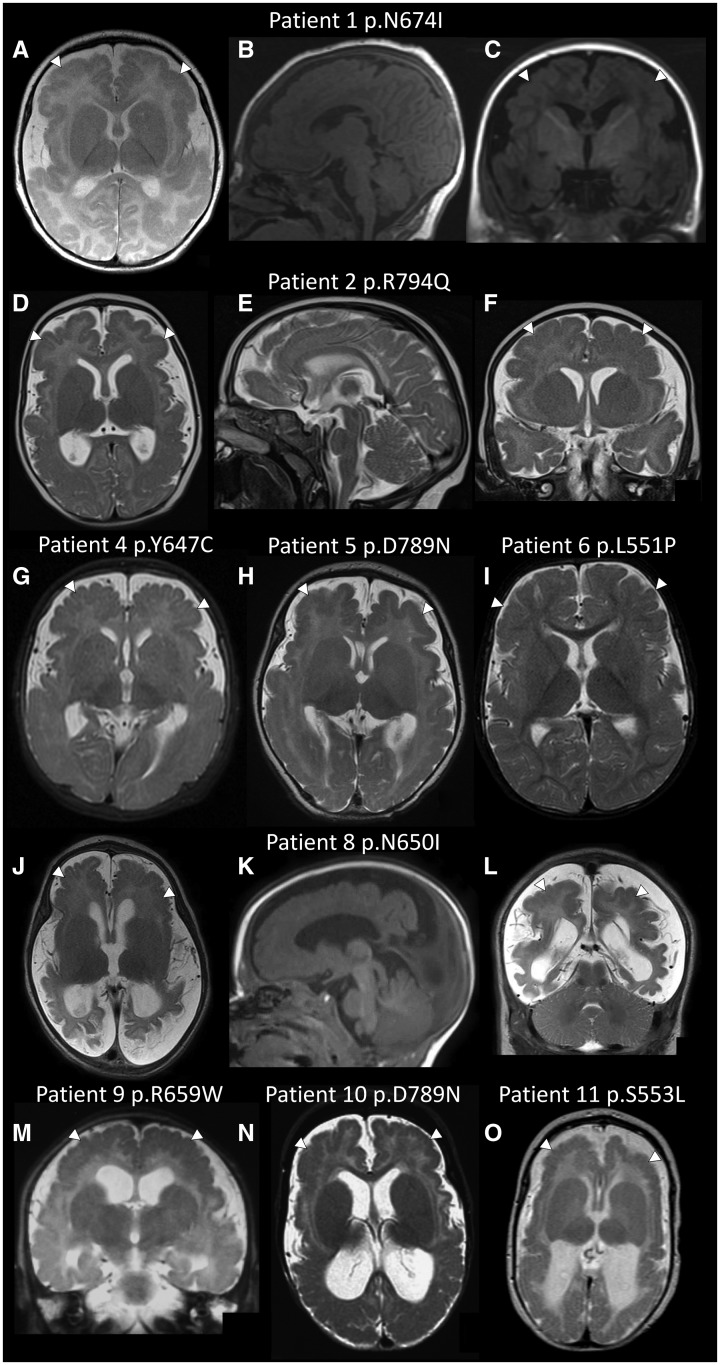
Polymicrogyria in patients with *GRIN1* mutations. Axial, midline sagittal and coronal brain magnetic resonance images for Patient 1 at age 2 months (A–C) and Patient 2 at age 5 months (D–F); axial magnetic resonance images for Patient 4 at age 3 months (G), Patient 5 at age 6 weeks (H) and Patient 6 at age 8 months (I); axial, sagittal and coronal images for Patient 8 at age 3 months (J–L); a coronal image for Patient 9 at age 4 months (M); axial images from Patient 10 at age 8 months (N) and Patient 11 at age 2 months (O). Images B, C and K are T_1_-weighted. All other images are T_2_-weighted. The images demonstrate bilateral extensive polymicrogyria (white arrows) more severe anteriorly. Note the increased extra-axial spaces and enlarged lateral ventricles (in most images apart from I) suggesting cerebral volume loss.
